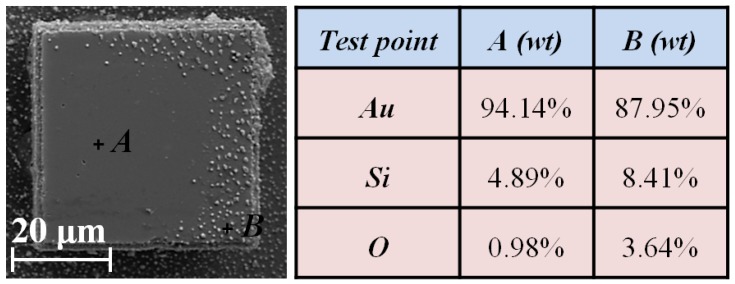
Energy dispersive X-ray test for Ti/Pt/Au mould after TEM.
